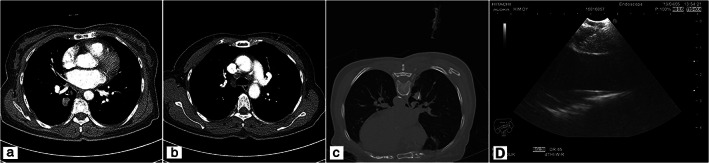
(a, b) Computed tomography (CT) scan showed a solitary pulmonary nodule in the right lower lobe and enlarged right lower paratracheal lymph nodes. (c) Computed tomography (CT)‐guided transthoracic needle biopsy. (d) Endobronchial ultrasonography‐guided transbronchial needle aspiration (EBUS‐TBNA)
